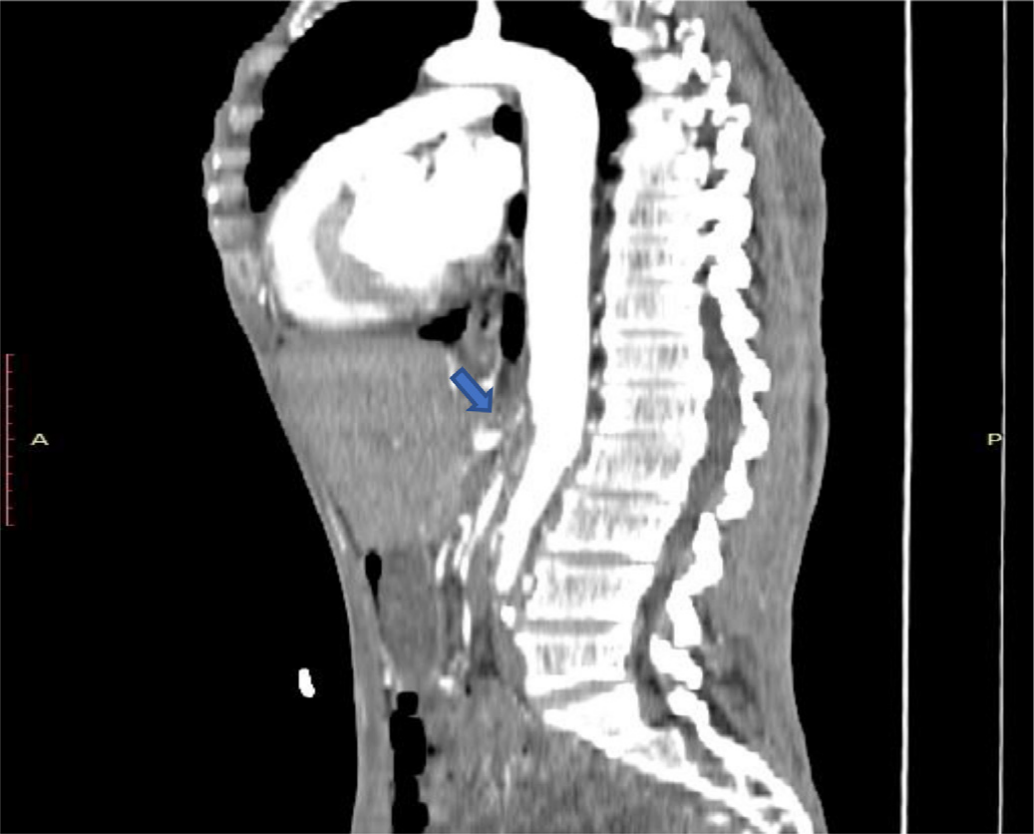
SMA total occlusion on the sagittal view of CT Angiography with arterial phase.
